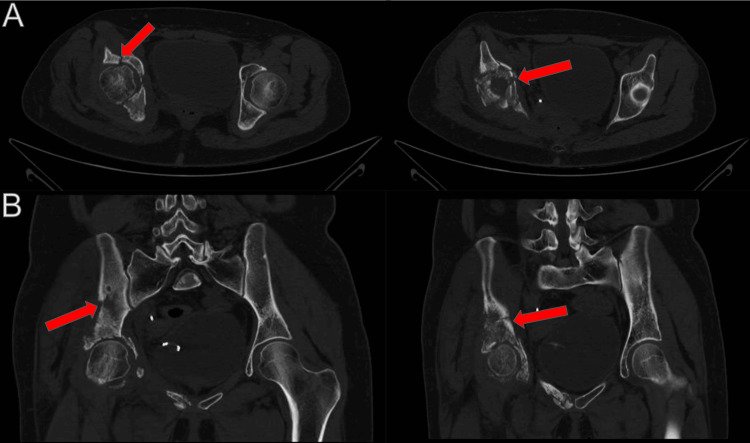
Contrast-enhanced CT of the pelvis Contrast-enhanced pelvic CT: axial views (A) and coronal views (B) showing extensive bone destruction involving the acetabular floor, supraacetabular area, and anterior wall and column (red arrows).
